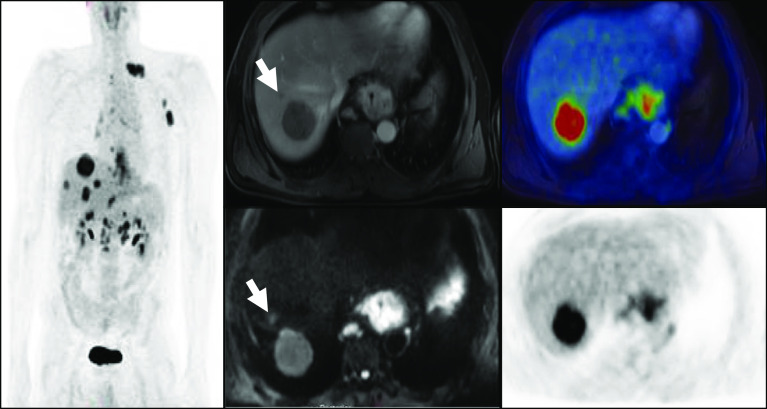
Oesophageal adenocarcinoma with additional liver metastases demonstrated by MRI. A non-FDG avid subcentimetre metastasis (highlighted by arrow) is demonstrated on diffusion-weighted and post-contrast-enhanced MRI, which was not detected by 18F-FDG PET/CT or contrast-enhanced CT
